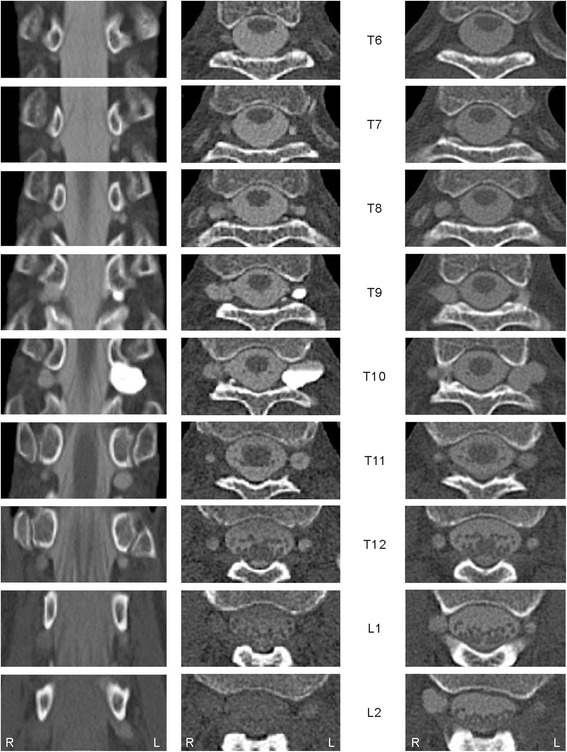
Computed tomography immediately after contrast medium injection (*left* and *middle* images) and 3 h later (*right* images). Two cysts at left T9 and T10 showed much higher intensity than the other cysts immediately after contrast medium injection
